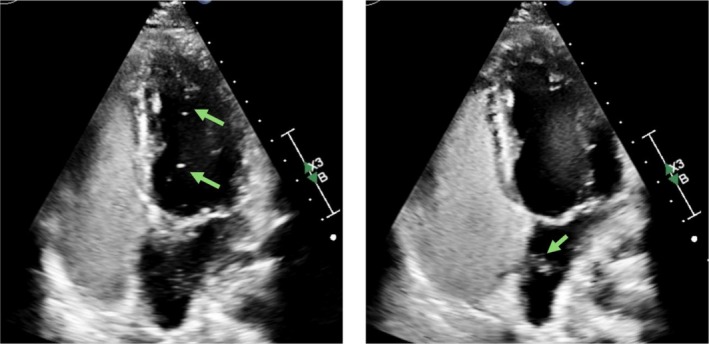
Bubble study demonstrating passage of bubbles into the left heart (left) via right‐to‐left shunting through the PFO (right) on transthoracic echocardiogram.
